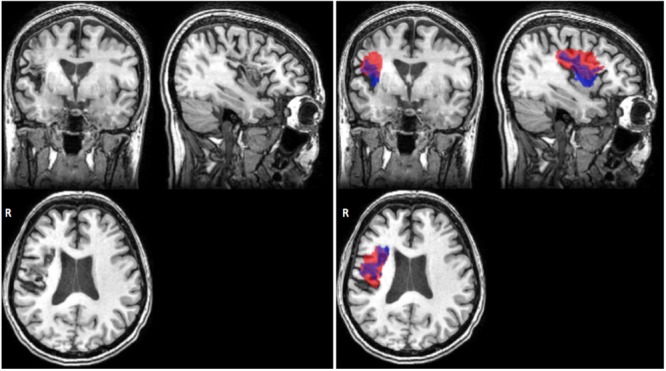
Representative case of automated versus manual lesion segmentation. Left, an individual’s T1w anatomical MRI; Right, the manual lesion mask in blue overlaid on automated lesion mask produced by the LINDA algorithm, in red (Pustina et al., 2016). DC, 0.57 for this lesion.
